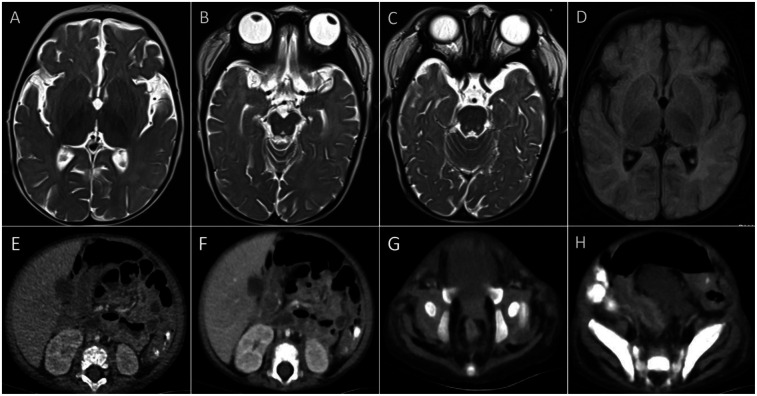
Imagines of CT and MRI. (A–D) Brain MRI showed asymmetric signals in the midbrain, pontine, bilateral lateral ventricle triangle and posterior horn. (E–H) Abdominal CT revealed thickening and strengthening of the intestinal wall and thickening of the vessels in the colon's hepatocele wall.
